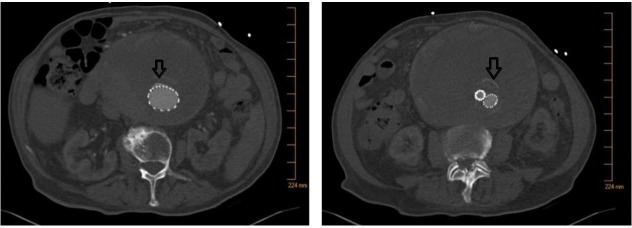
Axial computed tomographic angiography of an abdominal aortic aneurysm greater than 17 cm with arrow showing the endoleak
